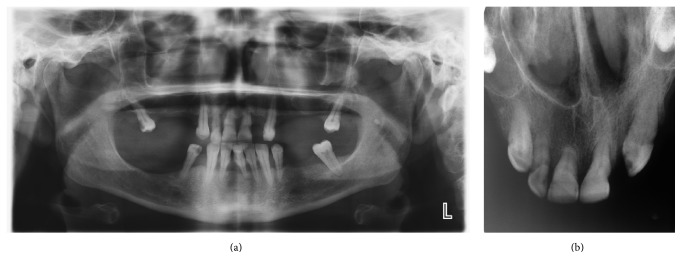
Patient's panoramic (a) and occlusal (b) radiographies show no bone changes on apical region of upper incisor teeth.
